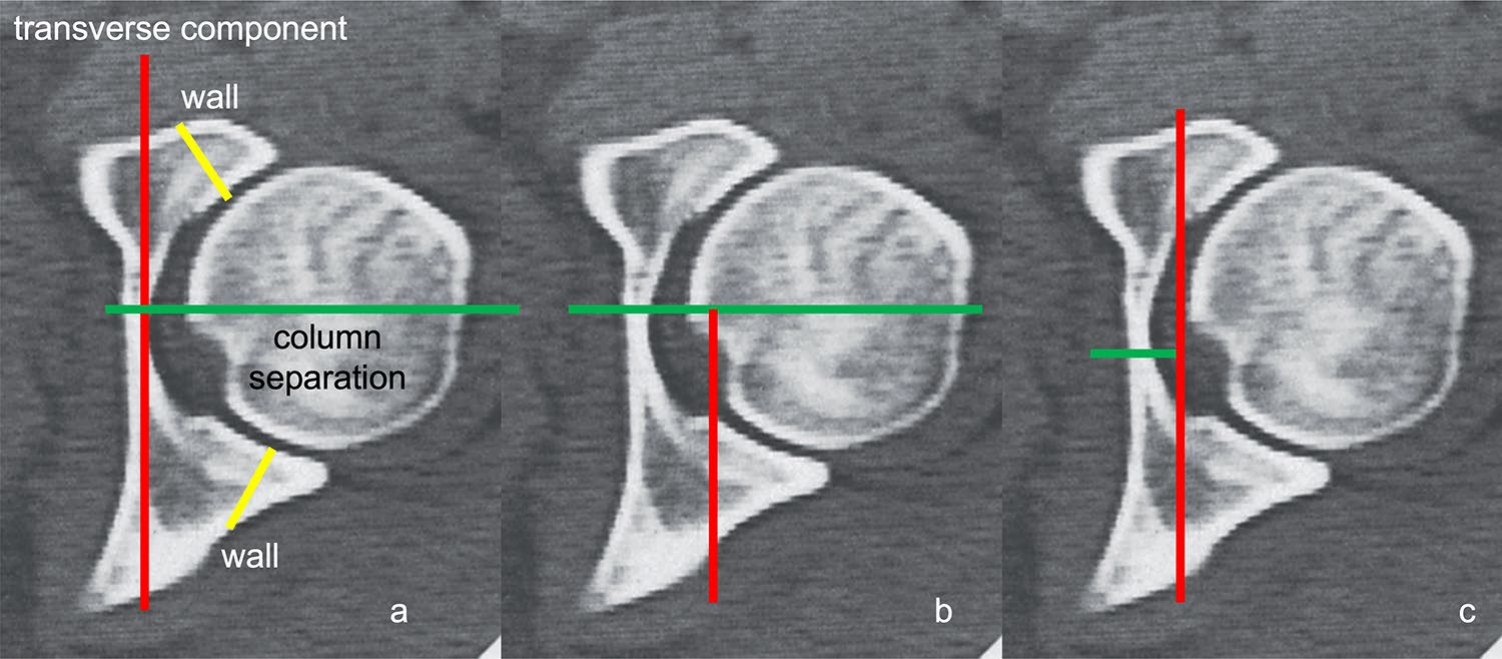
Basic fracture orientations on axial CT-views (a). Typical T-letter indicating and ACPHT fracture (b) and rotated T-letter in T-shaped fractures (c)
